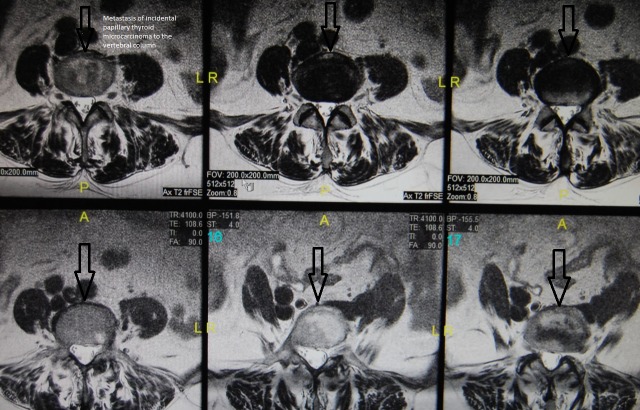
MRI transverse scan of the vertebral column showing a low signal area (arrows) of ITC metastasis in a 61-year-old female.
